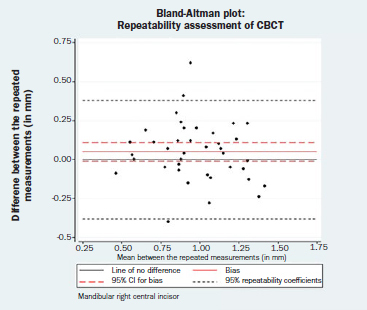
Bland-Altman plot: repeatability assessment for CBCT measurements, mandibular right central incisor.
